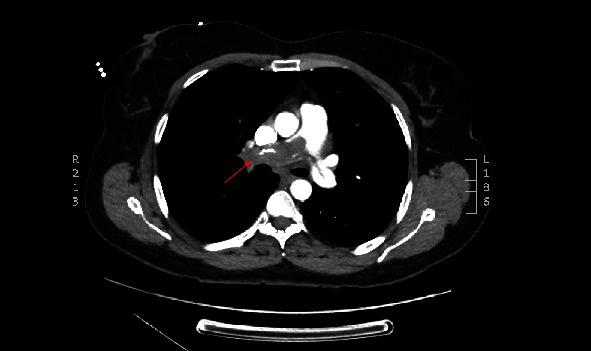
Transverse view of contrast-enhanced computed tomography images demonstrating a pulmonary embolism with subocclusion of the right pulmonary artery with extension to the left pulmonary artery and truncus pulmonalis.
